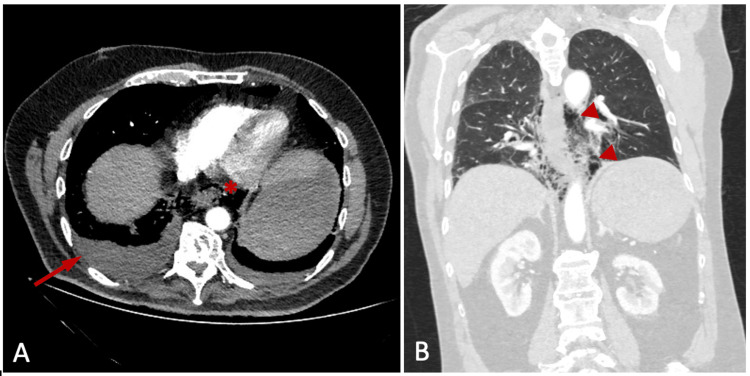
Chest CT scan findings in Boerhaave syndrome. (A) Axial plane which shows the free mediastinal air (*) and right pleural effusion (arrow); (B) coronal plane showing the extent of pneumomediastinum (arrowhead).
